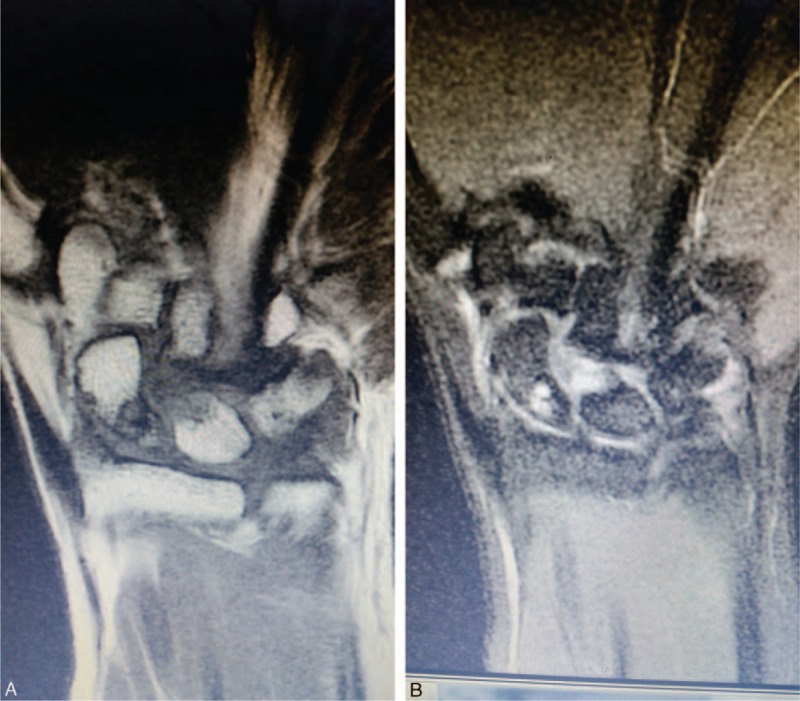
T1 (A) and T2 (B) weighted coronal cross section of the preoperative magnetic resonance imaging shows a bone cyst of 0.5 cm at proximal pole of scaphoid.
